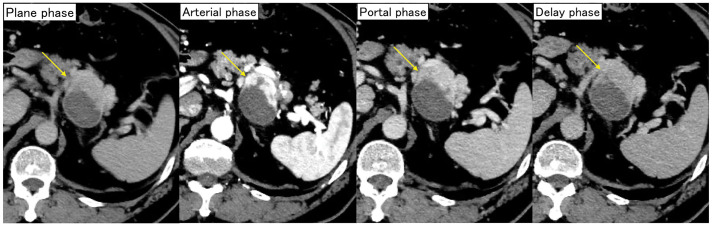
Dynamic-enhanced CT scan findings 2 months after the onset of abdominal pain. A CT scan showed a 55 × 42-mm mass on the dorsal side of the pancreas, and a marked contrast enhancement was observed in the ventral area of the tumor (arrows), but no noticeable contrast enhancement was observed in the dorsal area of the tumor.
